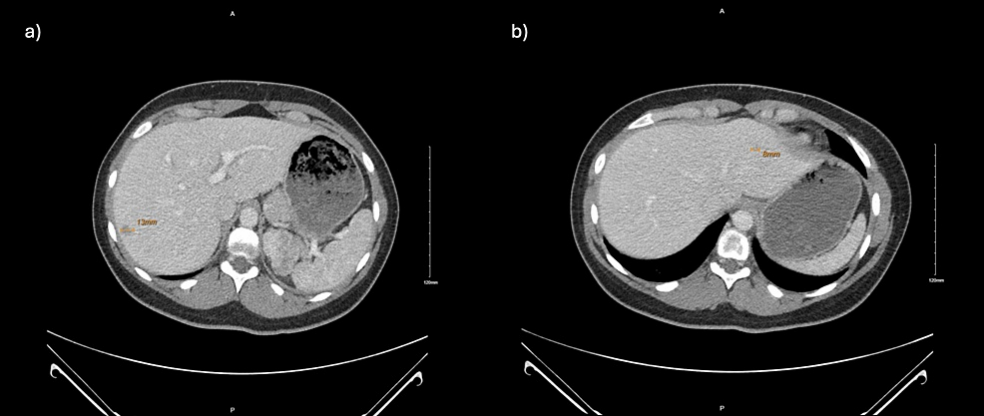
CT abdomen with and without contrast showing two hepatic lesions measuring 13 mm (a) and 8 mm (b)
